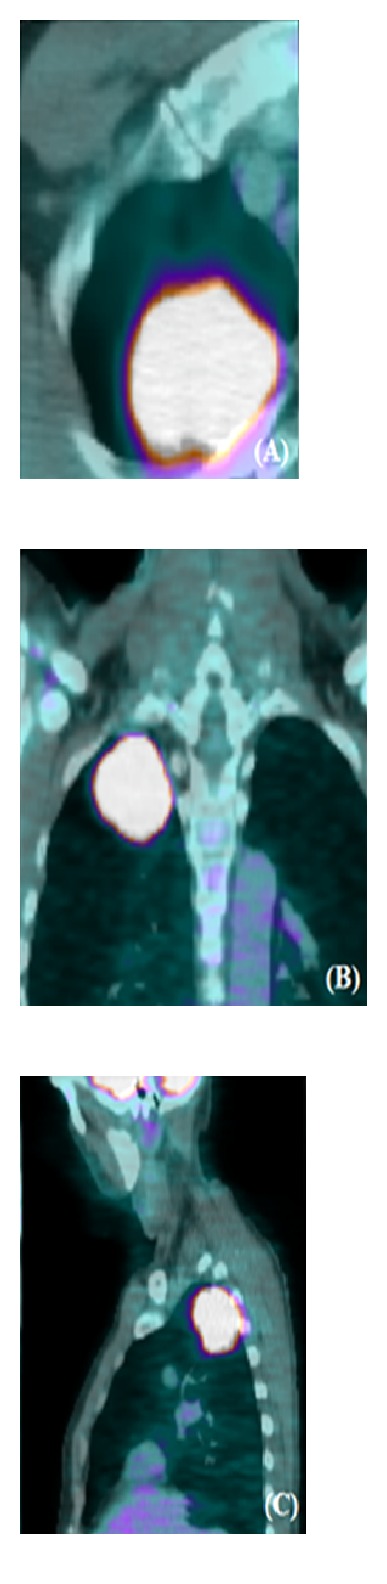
In PET-CT a high 18F-fluorodeoxyiglucose uptake confirms the neoplastic nature of a superior sulcus lesion. CT also allows a morphological study thanks to the possibility of multiplanar reformations on the axial (A), coronal (B), and sagittal planes (C).
